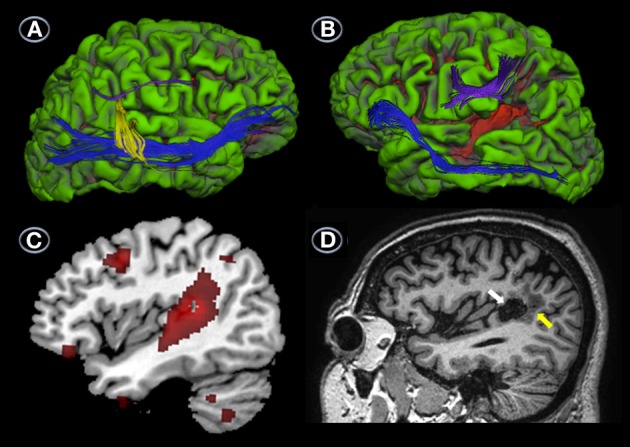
DTI, MRI, and PET. Uninflated surface of the right (A) and left hemispheres (B) (FreeSurfer reconstruction) of the patient with conduction aphasia (JGG) showing gyri cultured in green with sulci shown in red. DTI of right hemisphere perisylvian pathways superimposed on JJG anatomical MRI shows a complete lack of the direct temporo-frontal section of the AF in the right hemisphere. The indirect temporo-parietal (yellow) and parieto-frontal (purple) segments are present but very small. White matter tract reconstruction in the left hemisphere shows that the fronto-parietal segment of the AF (purple) was intact, but the temporo-parietal and temporo-frontal segments were unreconstructable, and seemingly destroyed by the lesion (B). The ventral stream (inferior frontal-occipital fasciculus/extreme capsule) was reconstructed successfully in both hemispheres (A,B). The surface image of the left hemisphere depicted in (B) also shows the infarction (red area) involving the posterior-superior and middle temporal gyri and part of the supramarginal gyrus. Parasaggital T_1_-weighted image shows a component of the infarction in the supramarginal gyrus (white arrow) surrounded by perinecrotic tissue (yellow arrow) (C). Parasaggital image of the ^18^FDG-PET (MRIcroN) showing an area of reduced metabolic activity in the left temporoparietal region (red) (D) which is slightly larger at the level of the posterior temporal gyrus than the area of infarction depicted in (C). Less voluminous foci of reduced metabolic activity (all in red) are also shown in the left middle frontal gyrus (Brodmann's area 6), lateral orbitofrontal cortex (Brodmann's area 11), inferior temporal gyrus (Brodmann's area 20), and cerebellum (D). See further details in Table 4.
